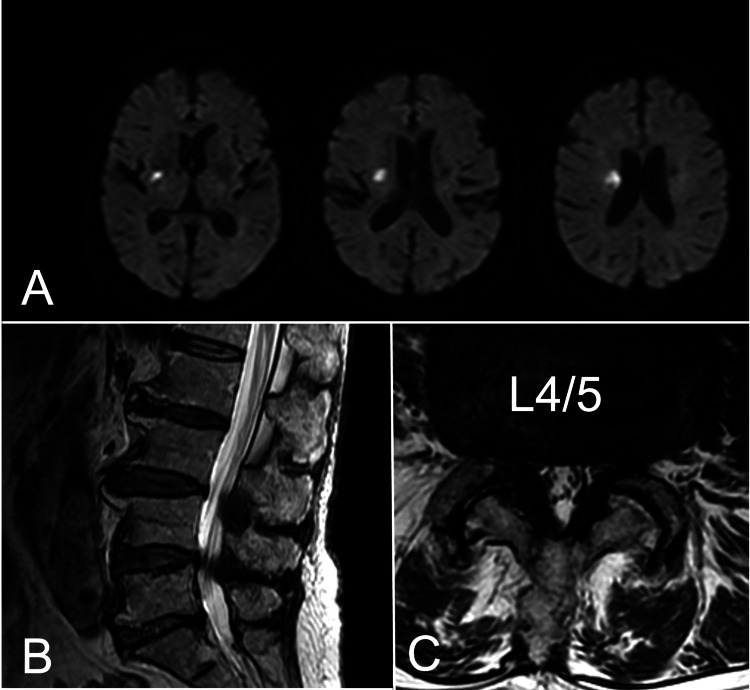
Initial head MRI and lumbar spine MRI after admission. (A) Diffusion-weighted brain MRI (axial images) performed on the day of admission. Note the small cerebral infarct in the right corona radiata. (B, C) T2-weighted lumbar spine MRI (B: sagittal image; C: axial image) showing lumbar spinal canal stenosis at L4/5.
